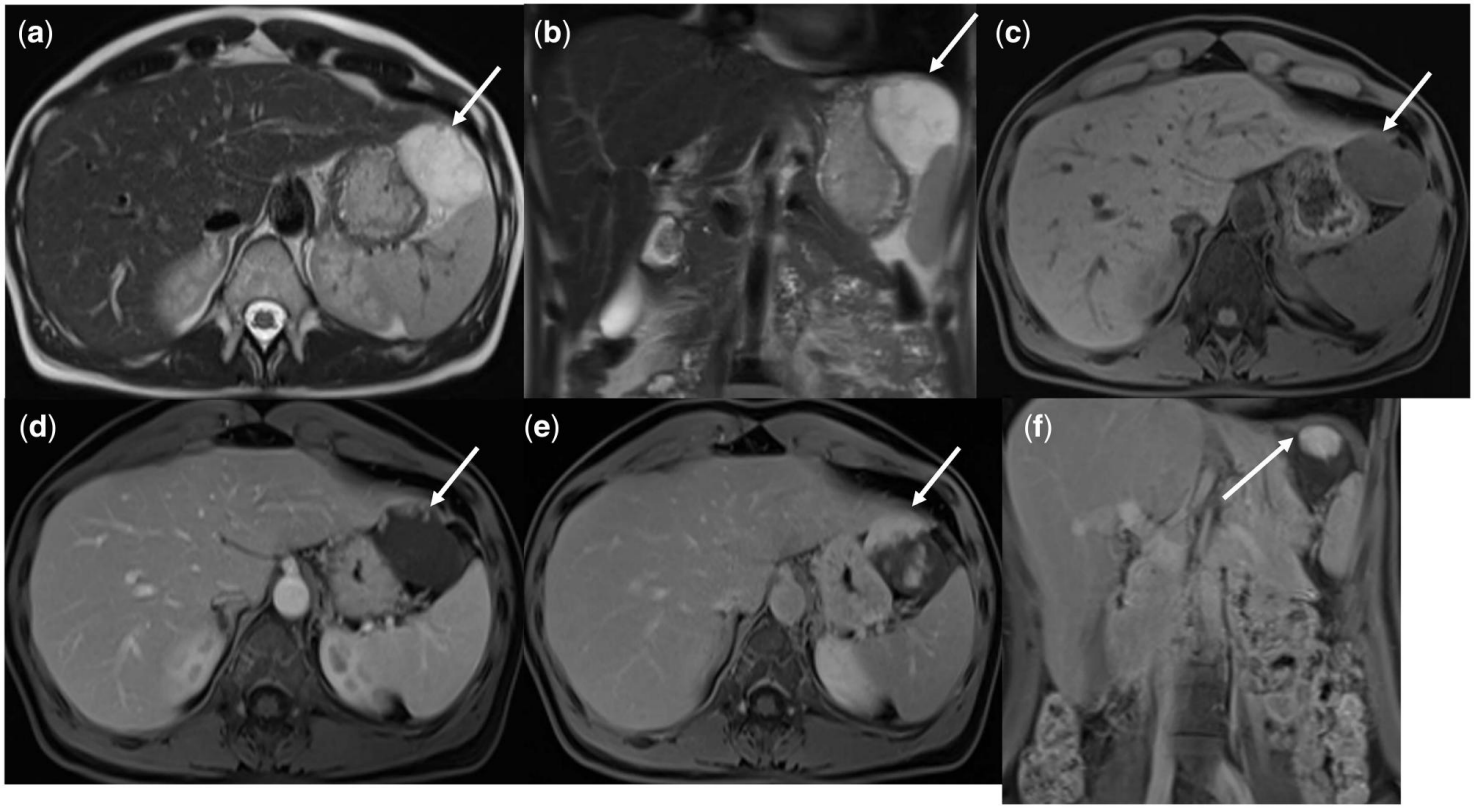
False Positive Finding on Screening WB-MRI. 54-year-old asymptomatic patient underwent screening whole-body MRI (WB-MRI) without IV contrast, including axial and coronal T2-weighted single-shot fast spin-echo (SSFSE) sequences (a, b) and T1-weighted gradient-recalled echo (GRE) sequence (c), which demonstrated a 4.2 cm T2 hyperintense mass in the left upper quadrant (arrows a, b, c), which was read as indeterminant and possibly representing a gastric mass, and a contrast enhanced abdomen MRI was recommended. Contrast enhanced MRI performed 7 days later with axial T1-weighted GRE during the late arterial (d) and portal venous (e) phases as well as coronal T1-weighted GRE during the portal venous phase (f) demonstrated that the left upper quadrant mass demonstrated peripheral nodular discontinuous enhancement progressing centripetally from late arterial to portal venous phase images (arrows, d, e, f), representing an exophytic segment II hepatic hemangioma.
